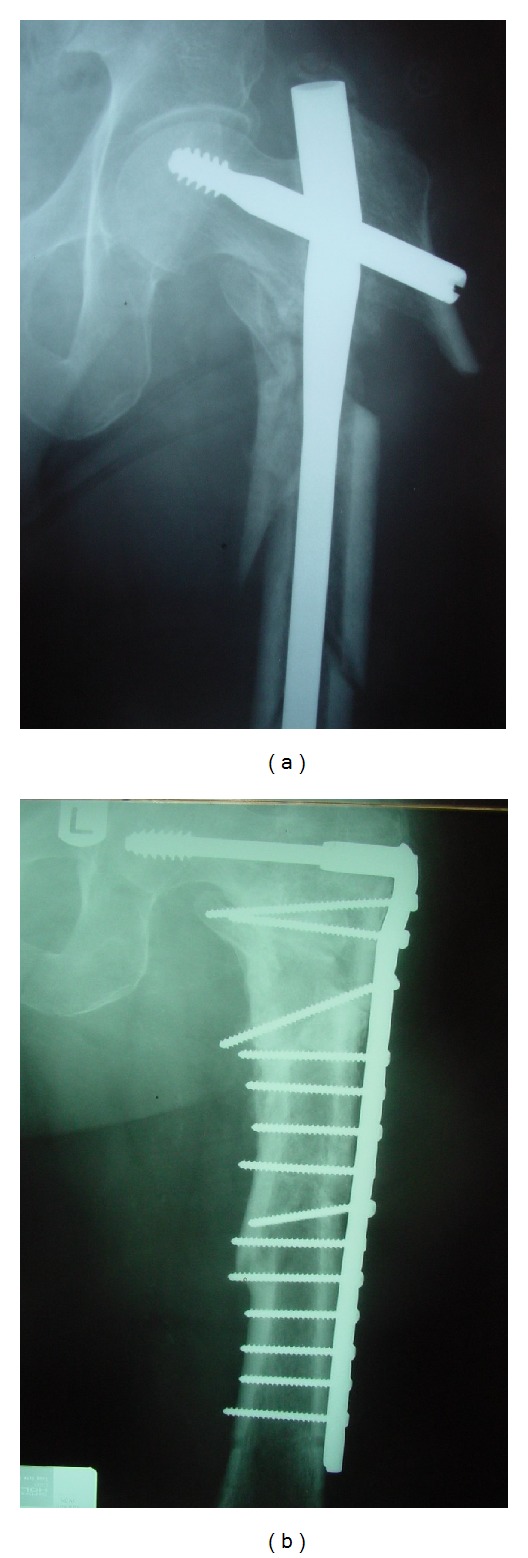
AP radiograph of a complex intersubtrochanteric fracture of femur, showing a broken long TGN at the junction of the nail with the lag screw (a). The nail was revised to DCS plate and the fracture healed at 4 months postoperatively (b).
